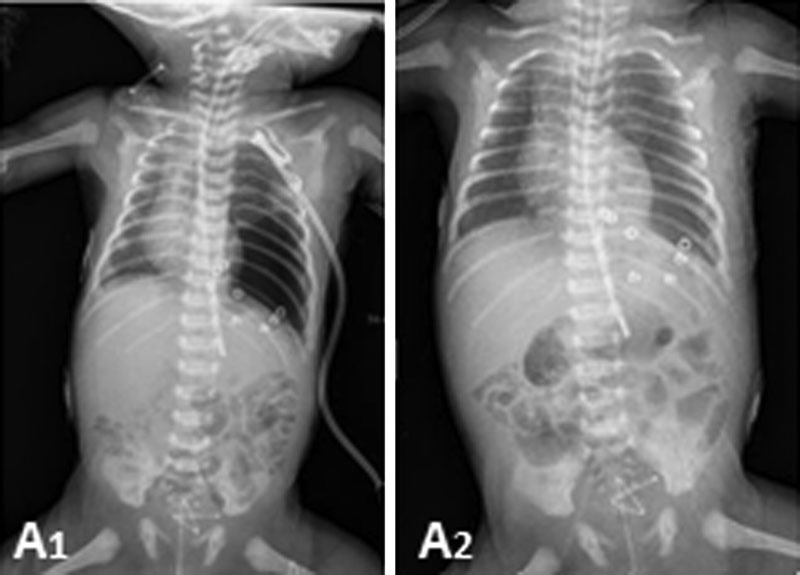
Postoperative chest radiograph: (A1) Immediate postoperative and (A2) Two days postoperatively. The size of the left lung gradually increased, and the mediastinal shift resolved.
